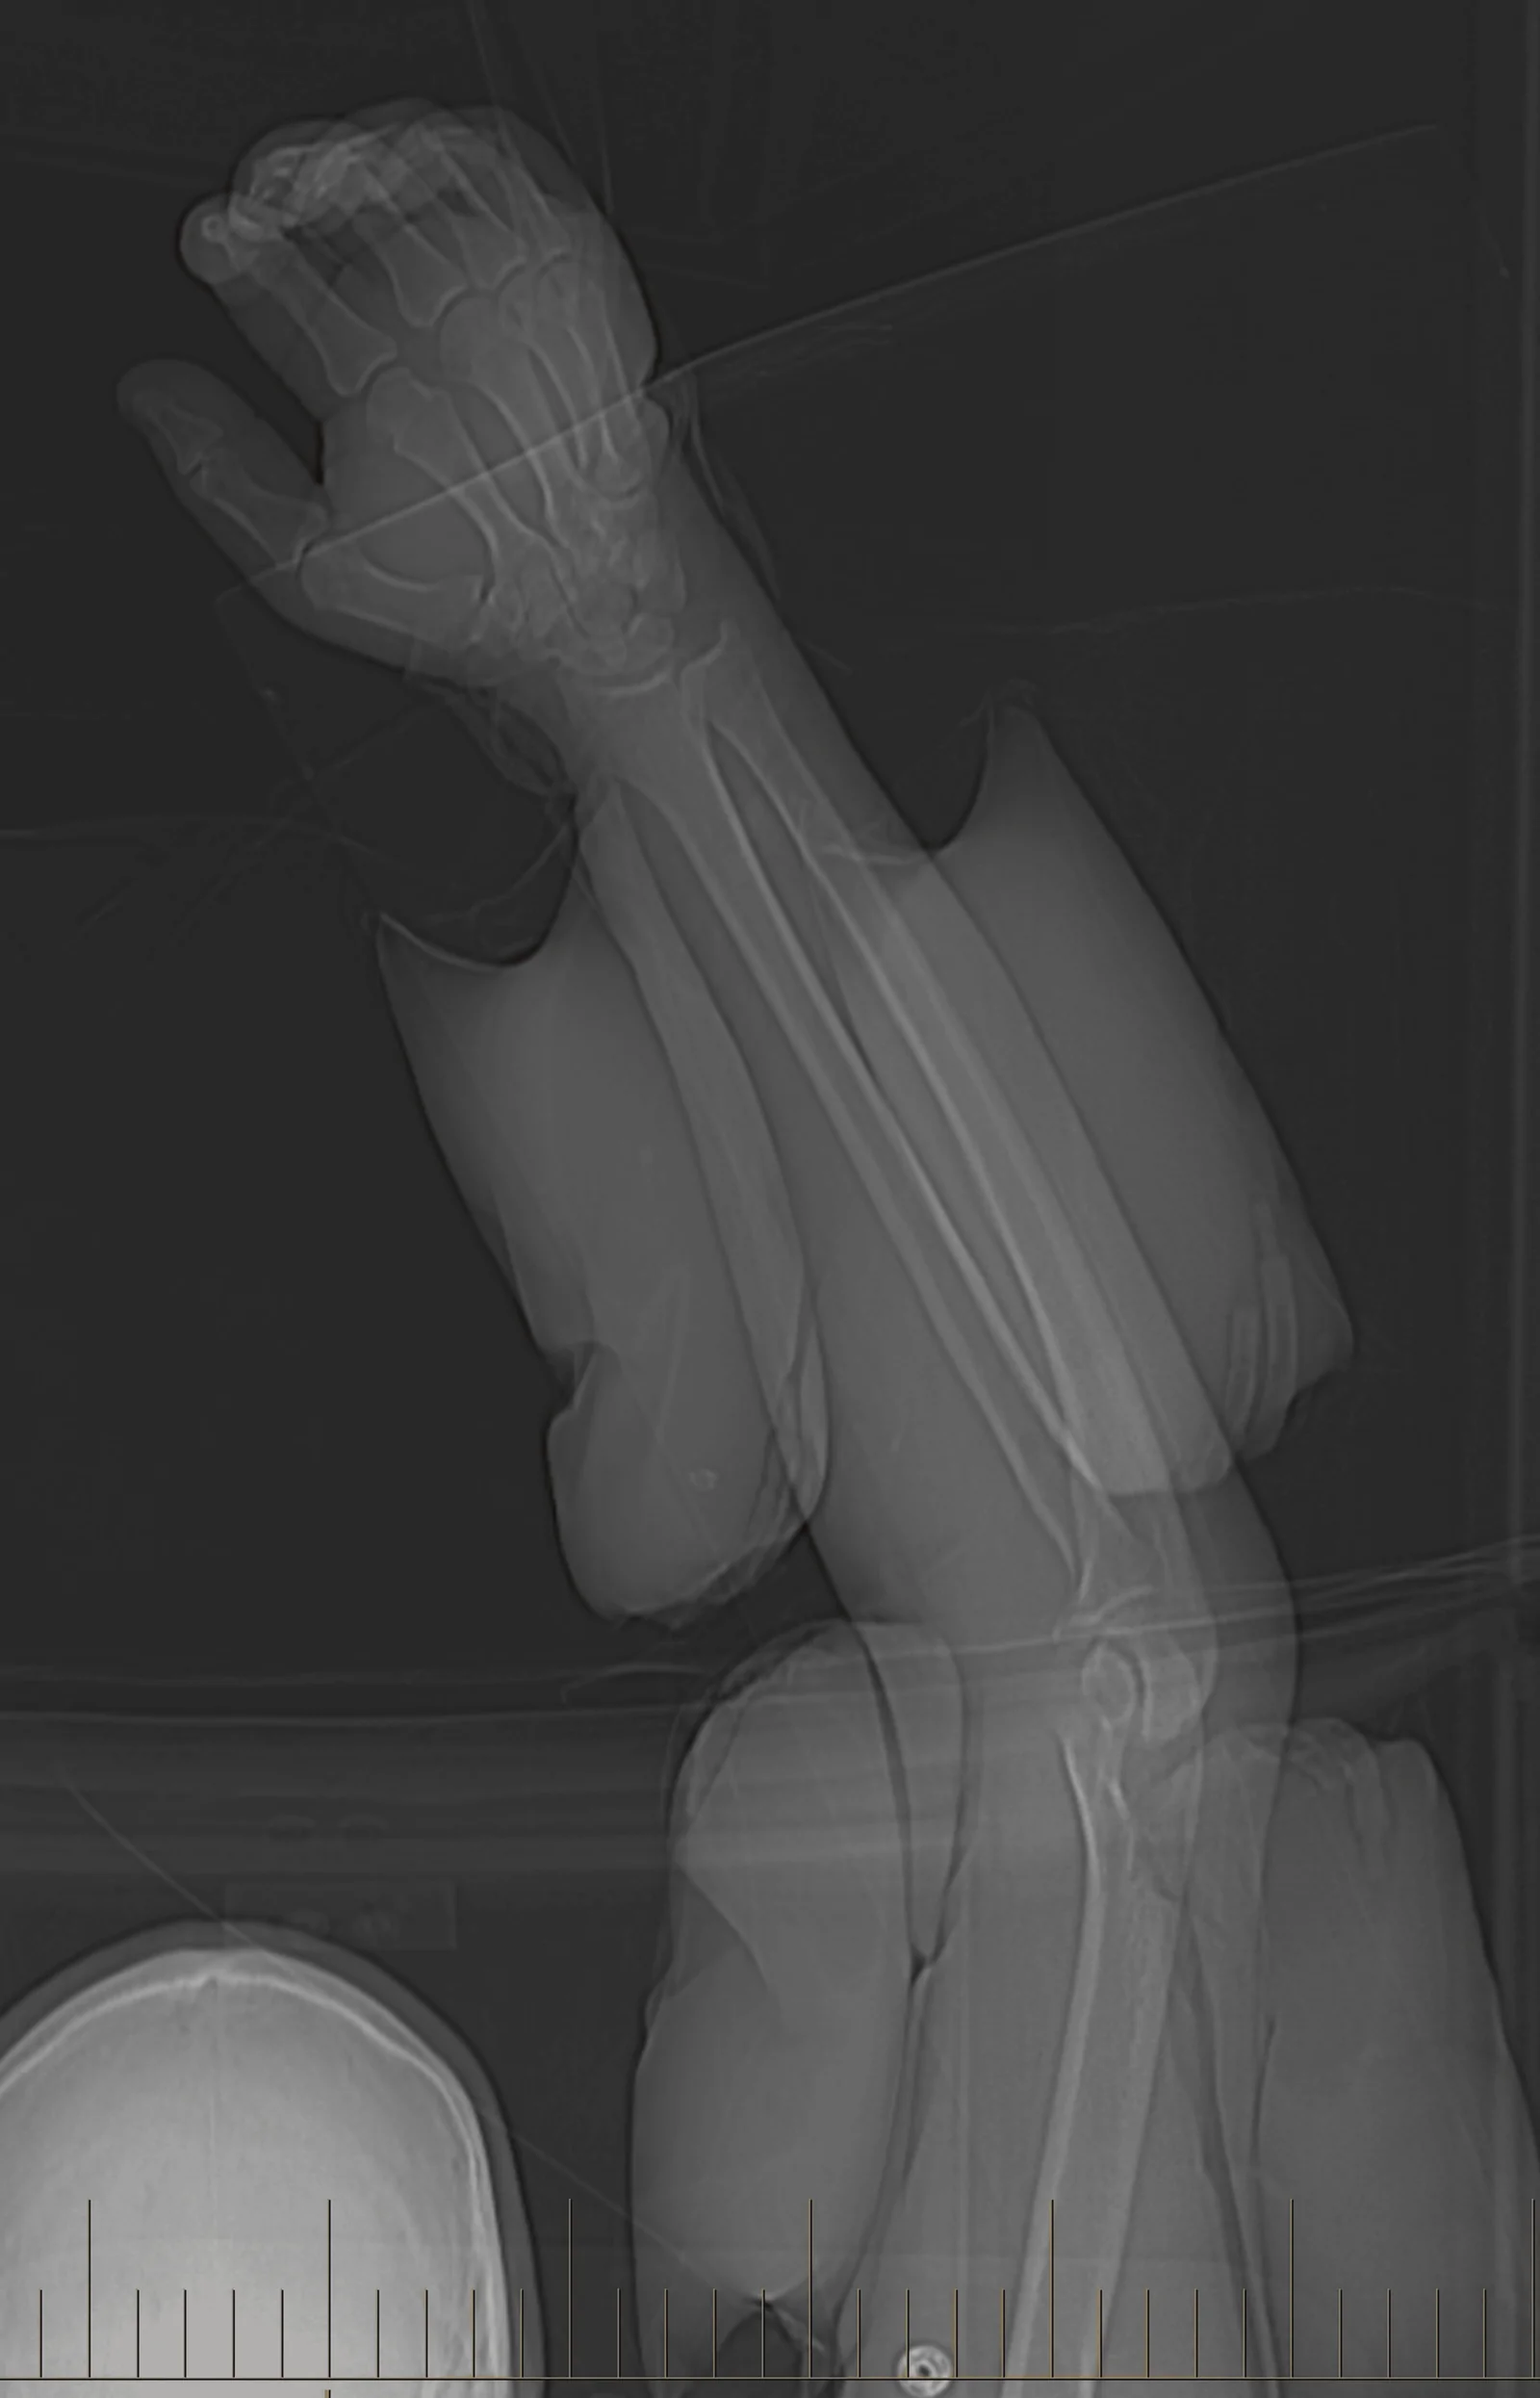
AP view X-ray of the right upper extremity showing no bony involvement
